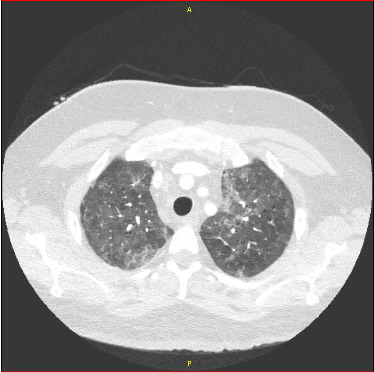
CT chest on the day of admission.
